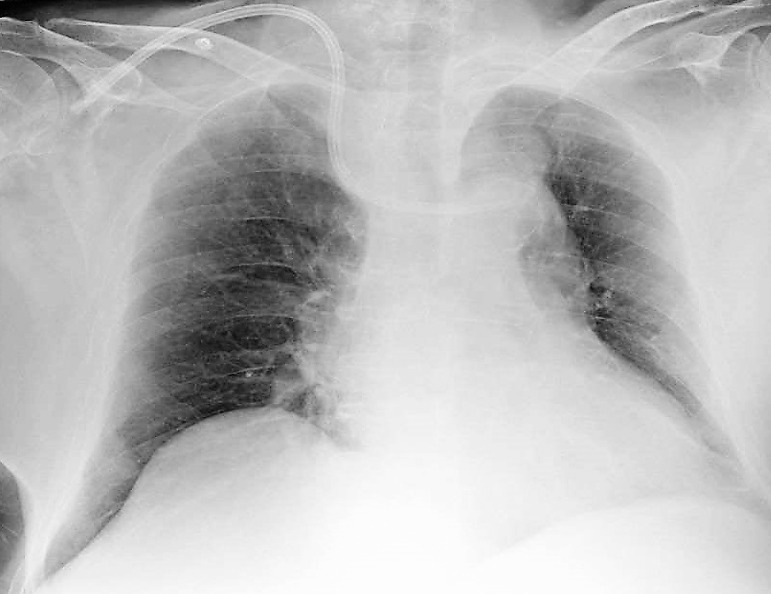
Anterior-posterior chest radiograph. The tip of the catheter is in a left sided position. There is widening of the mediastinum with a regular pale opacity above the aortic knob.
